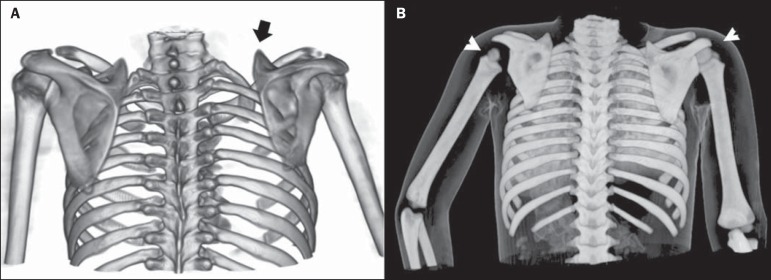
Examples of three-dimensional volume rendering of computed tomography
